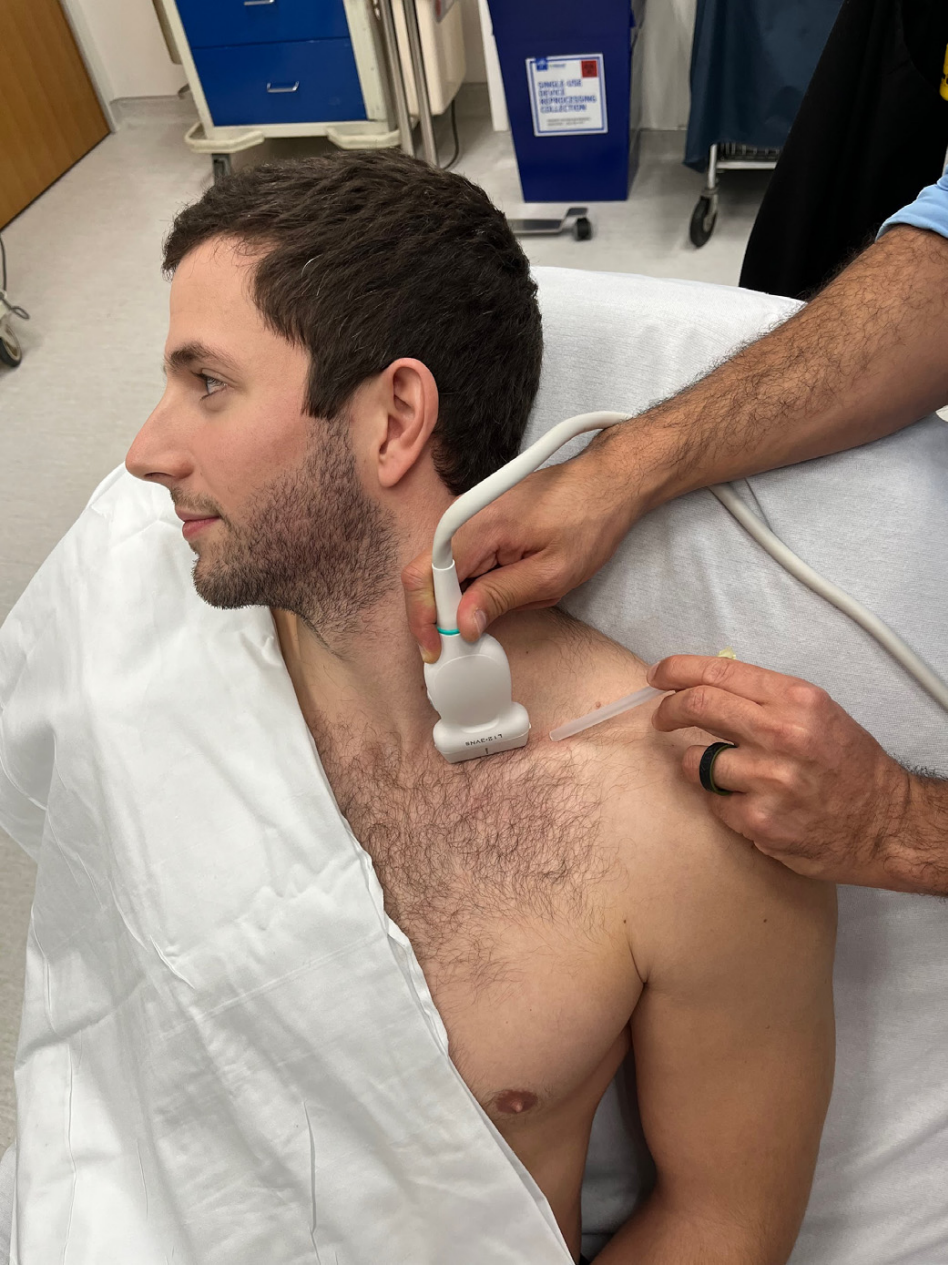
Ultrasound probe placement in the supraclavicular fossa to identify and anesthetize the supraclavicular brachial plexus. The needle is shown before introduction from a lateral to medial approach (shown here on a model patient).
